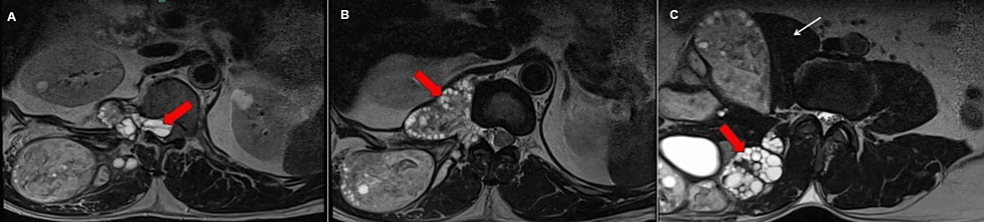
Magnetic resonance imaging of T2-weighted axial images of the abdomen and pelvis. (A) Intraspinal extension of the cysts (red block arrow). (B) Paraspinal muscle involvement with a pleuroperitoneal excursion along with multiple daughter cysts (red block arrow), causing mild scalloping of the adjacent vertebral body. (C) Multiple daughter cysts (red block arrow) are seen within the mother cyst in right quadratus lumborum and erector spinae muscles. Right psoas muscle (white arrow) is displaced anteromedially.
